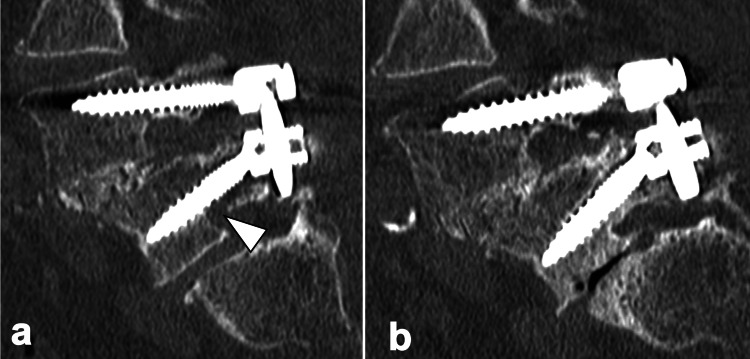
Sagittal computed tomography of the lumbar spine in Case 2 a. Sagittal CT image obtained 36 days postoperatively demonstrating bilateral L5 pedicle fractures. b. Sagittal CT image at 1.5 years postoperatively demonstrating complete bone union of the L5 pedicle fractures.
